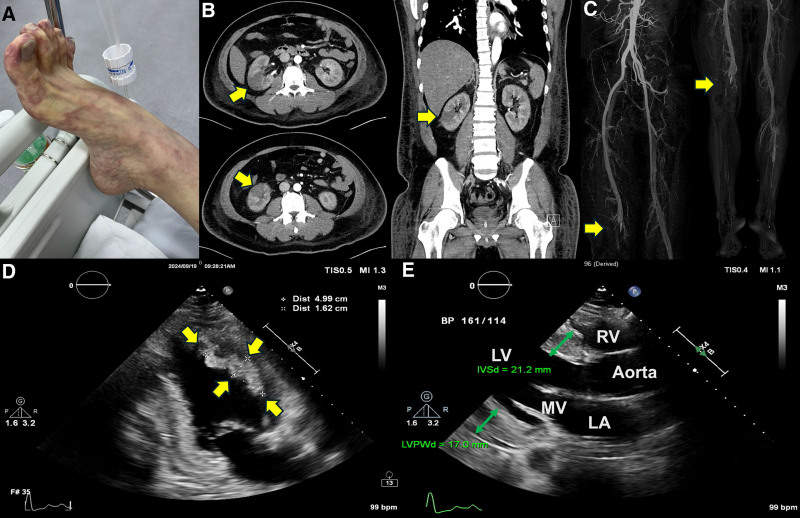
Initial patient condition. (A) Gross appearance, (B) CT angiography showing right renal infarction (arrow), (C) CT angiography showing total occlusion of the right distal superficial femoral artery (arrow), (D) echocardiography showing LV thrombus (arrows), and (E) echocardiography showing LV hypertrophy (green arrows). CT = computed tomography, LV = left ventricle.
